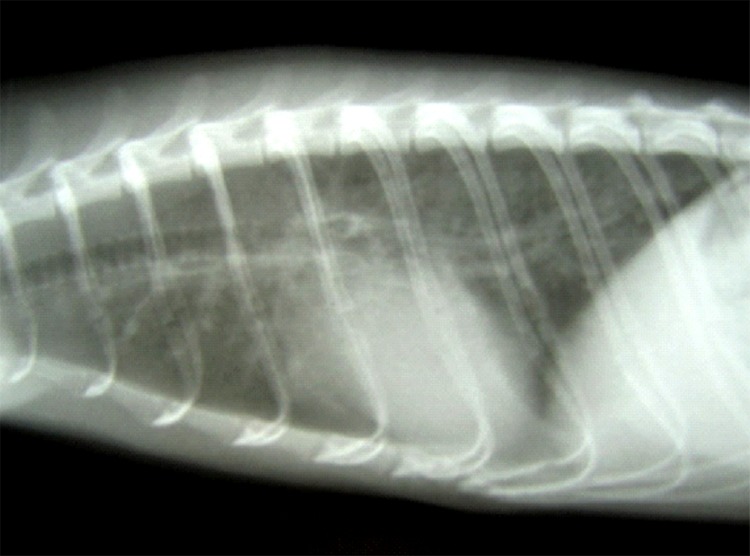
Lateral radiographic view of the thorax from a 2-year-old ferret with cough
